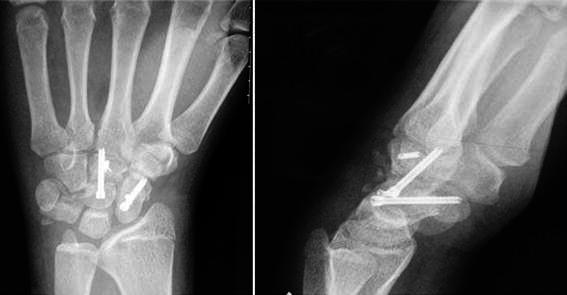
Radiographs at follow-up showing optimally reduced fractures of the scaphoid and capitate and reduction of the perilunate dislocation
